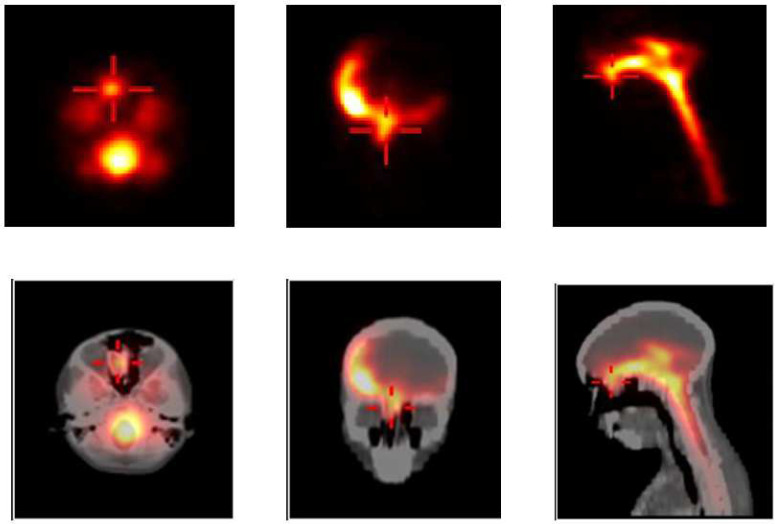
Planar static images (top row left: axial view; top row middle: coronal view; top row right: sagittal view) show an abnormal focal uptake in the frontal skull region as depicted by the cross. Bottom row: SPECT-CT views localising the uptake to the right ethmoid bone.
